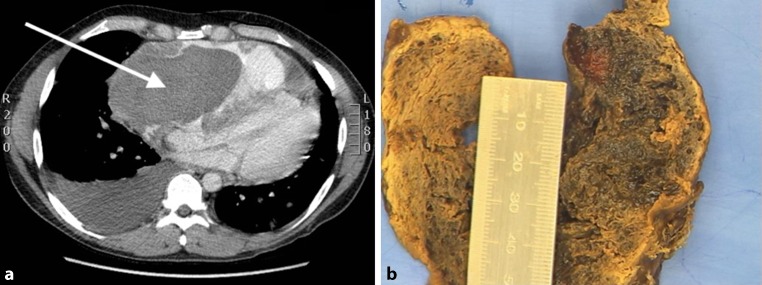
a Chest CT image with a very large extra-cardiac mass and 6 cm pericardial effusion with compression of the right atrium and right ventricle. Space-occupying lesion in the right atrium, diffuse lesion in both lungs and in the spleen. Pleural effusion right. b Macroscopic image of an angiosarcoma
